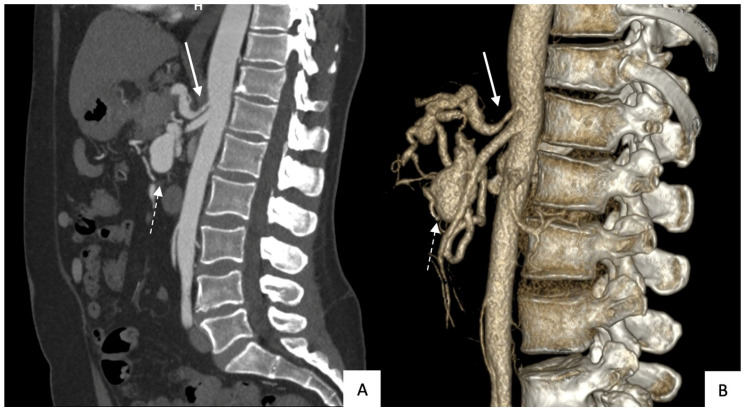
Computed tomography in a sagittal reconstruction (A) and with volume rendering (B) showing stenosis of the celiac-trunk ostium due to compression by the median arcuate ligament (solid arrow) and an aneurysm of the pancreaticoduodenal artery (dotted arrow).
